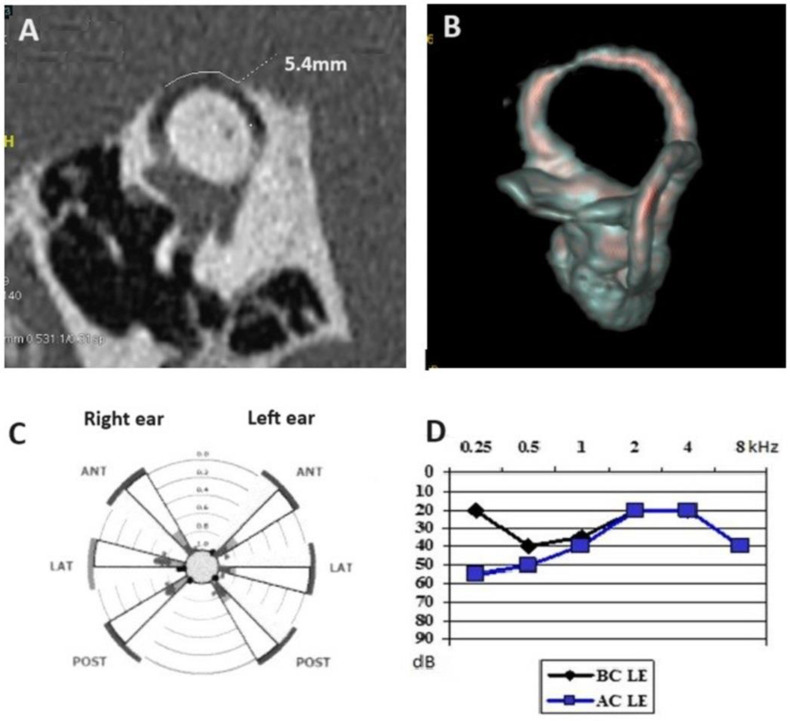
(A) High-resolution computed tomography (HRCT) scan of the petrous bone showing a 5.4 mm left-sided large dehiscence of the SSC—Pöschl incidence. (B) 3D HR MRI of the labyrinth confirming a narrowing at 0.5 mm of the SSC (for a normal diameter of about 1.2 mm)—multiaxial 3D plane view; (C) Video Head Impulse test showing normal gains for all SCC. (D) Left-sided mixed hearing loss with an air-bone gap of 40 dB at 0.25 kHz. SSC, superior semicircular canal. 3D HR MRI, three-dimensional high-resolution magnetic resonance imaging.
